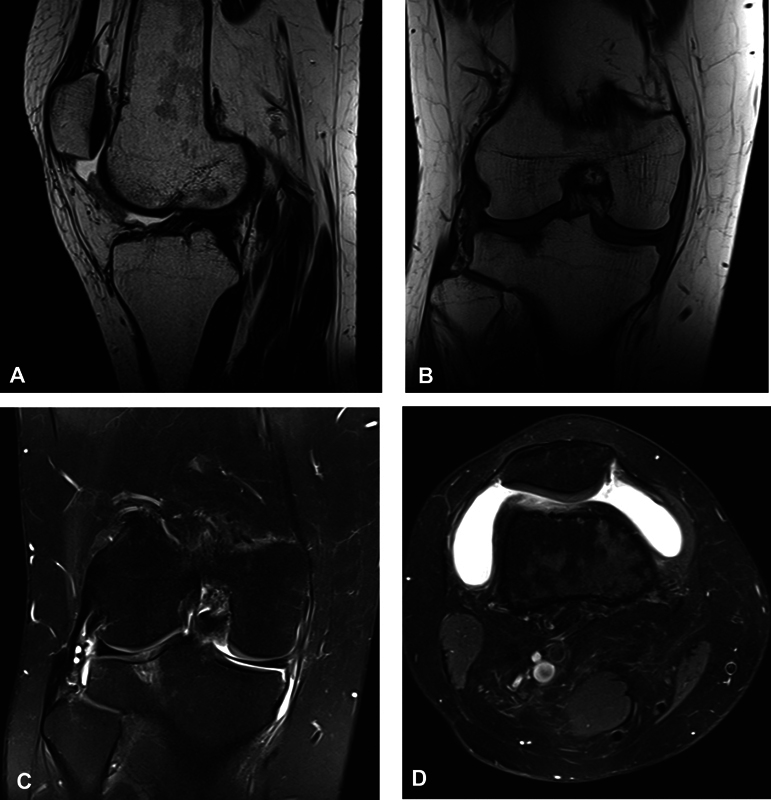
Magnetic resonance images.
